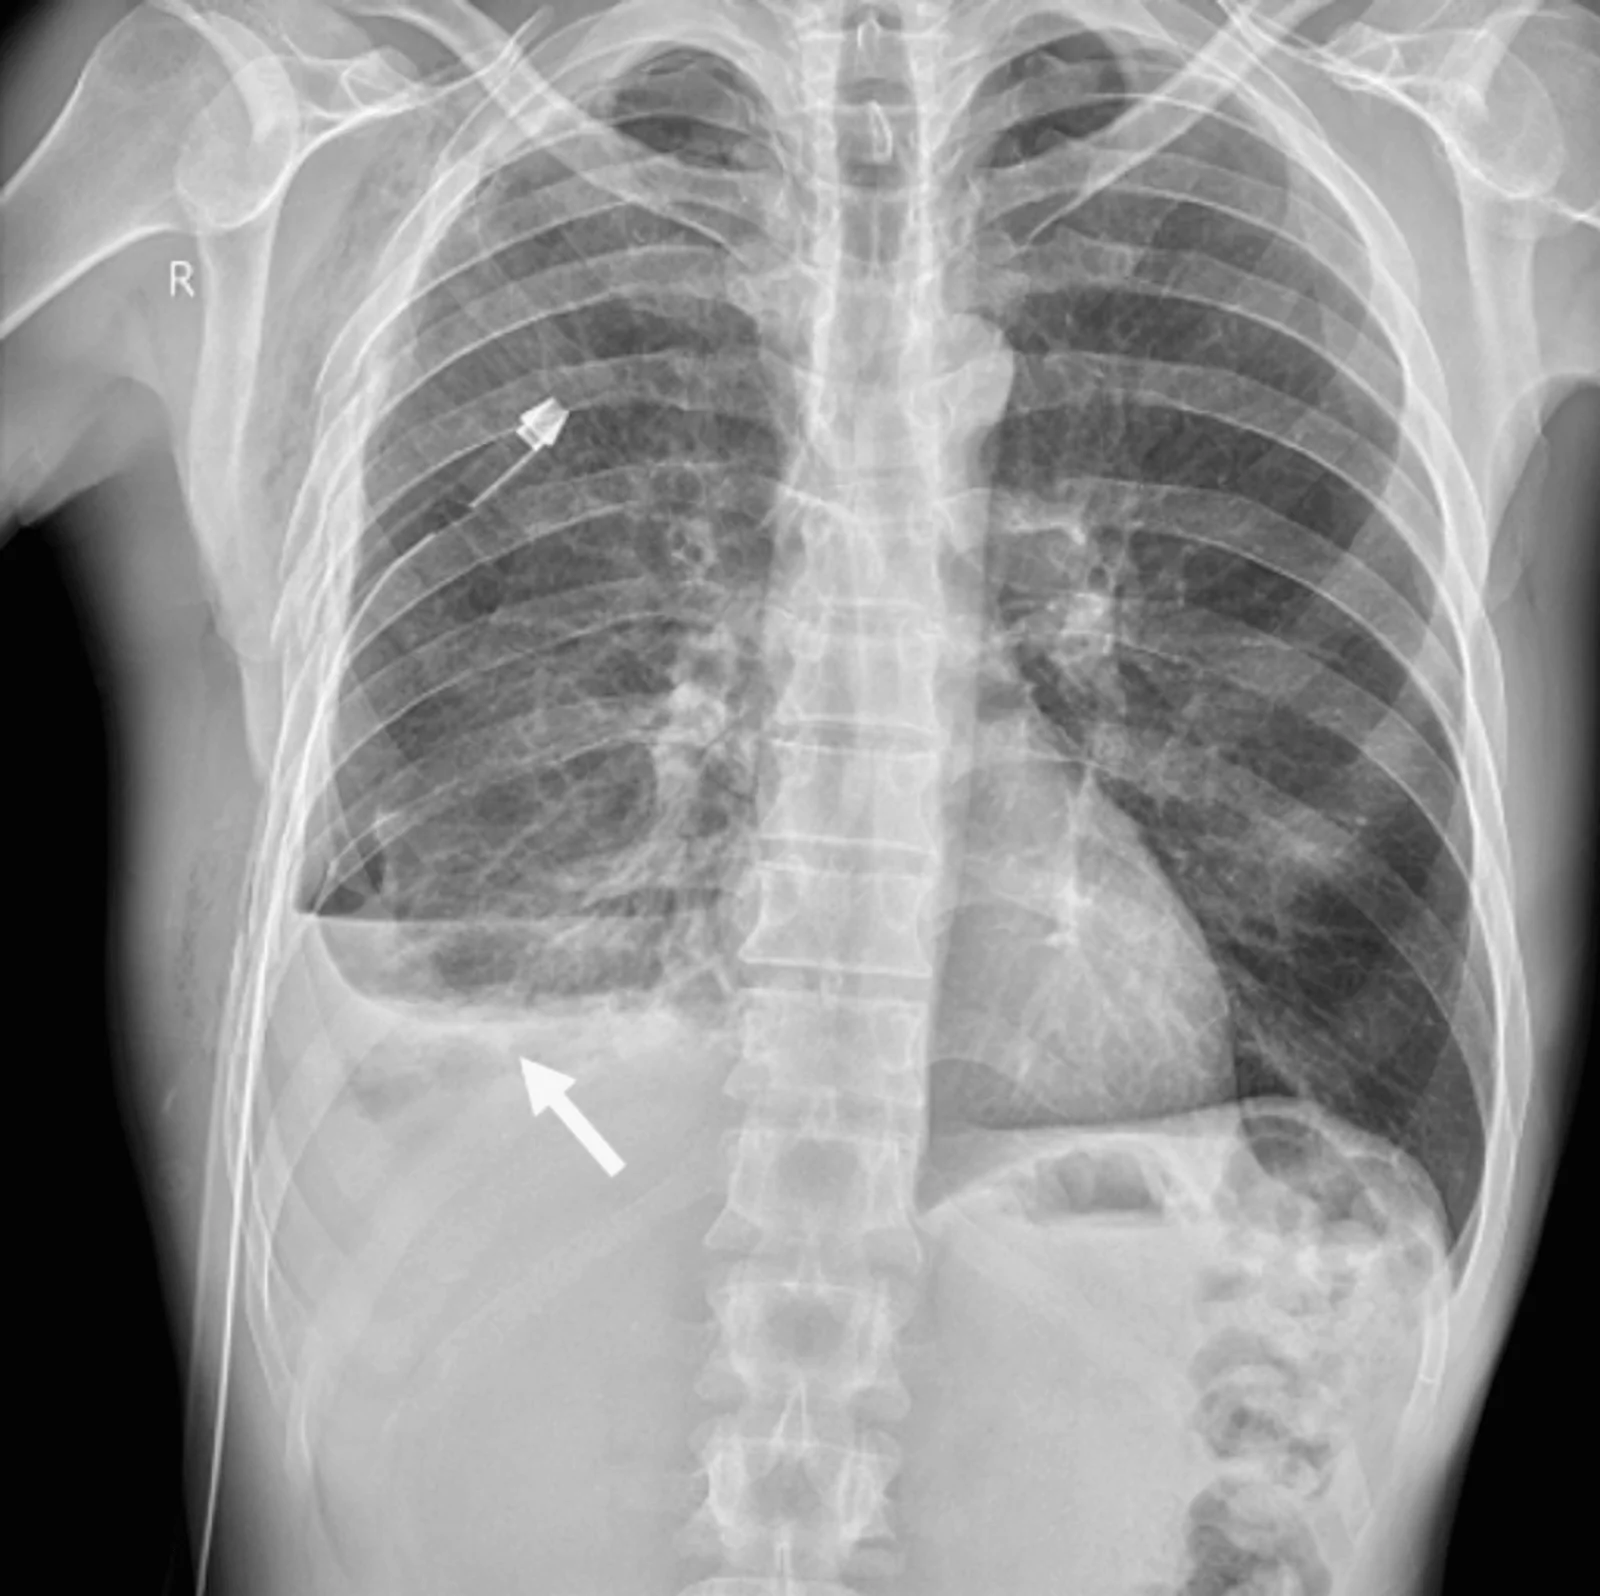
Follow-up anteroposterior chest radiograph on day two of admission. The image demonstrates ongoing clinical progress following right-sided chest tube placement, showing progressive re-expansion of the right lung parenchyma. Compared to the previous radiograph, the residual pleural fluid at the right lung base shows a further decrease in the volume of the hemothorax (white arrow).
